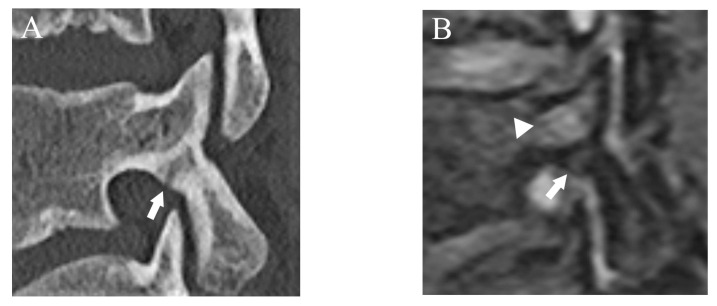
The case that lower fracture grade was assigned by DESS than CT. (A) CT suggests an incomplete fracture which was consistent with grade 2 (arrow). (B) DESS shows a subtle high signal in the cortex of the pars interarticularis and evaluated as grade 1 (arrow). BME is also detected (arrowhead).
